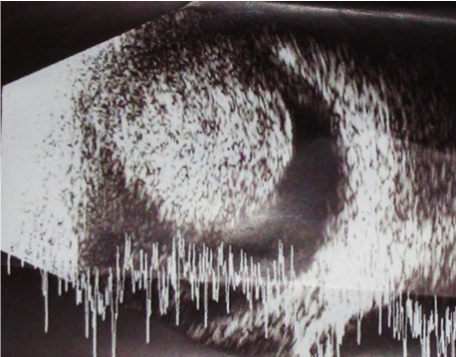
Ultrasonography showing a mushroom-shaped, elevated, solid lesion with regular internal structure and low to moderate internal reflectivity suggestive of choroidal melanoma
